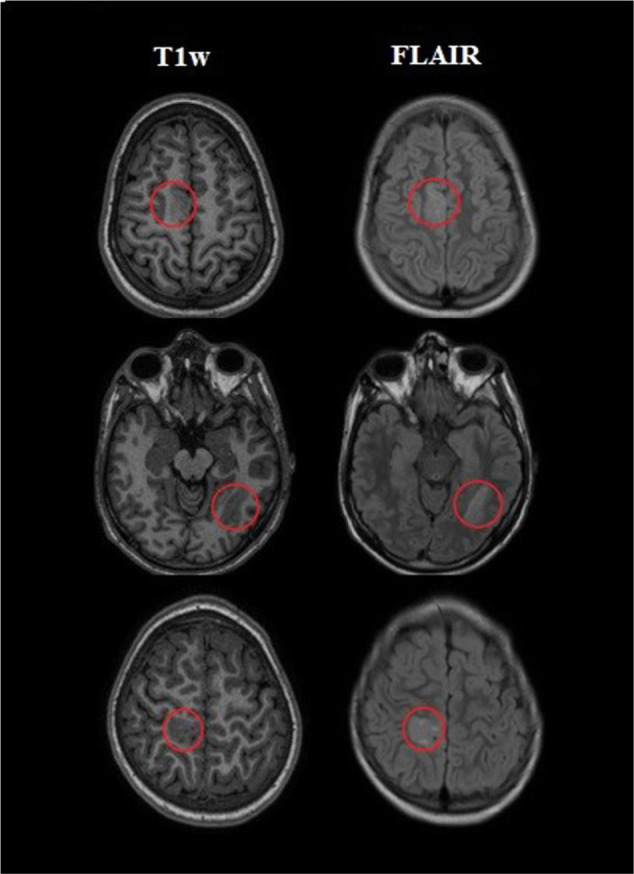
Examples of three patients with a labeled lesion. The first column on the left indicated the presurgical three-dimensional T1-weighted (T1w) images which were utilized as input for the processing. The next column revealed T2-weighted fluid-attenuated inversion recovery (FLAIR) images on the same slice.
